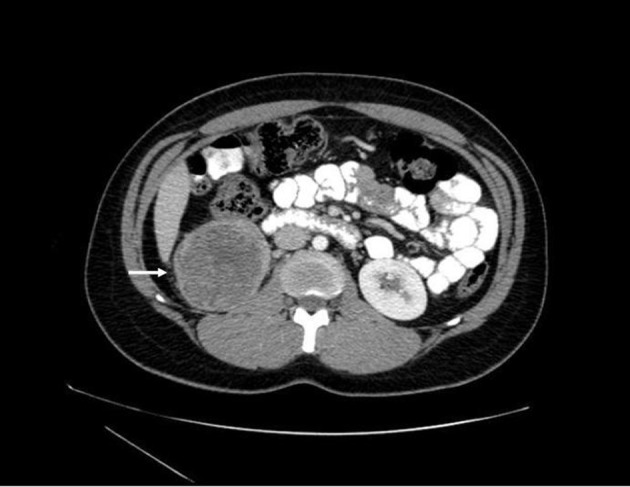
Coronal CT (with oral and intravenous contrast enhancement) showing large heterogeneously enhancing mass replacing lower pole of the right kidney.
